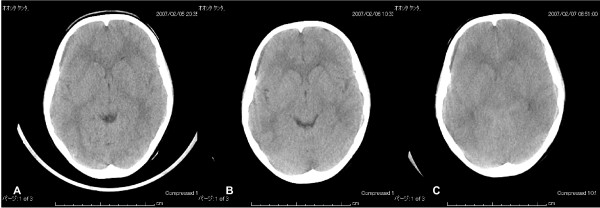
The changes of CT findings at the level of the basal ganglia of Case 1. A: CT scan on admission showing slight edema without loss of gray or white matter differentiation. B: CT scan 24 hours after admission showing definite basal cistern and cerebral sulci. C: CT scan 48 hours later showing severe edema with vanishing basal cisternae and loss of gray and white matter differentiation.
